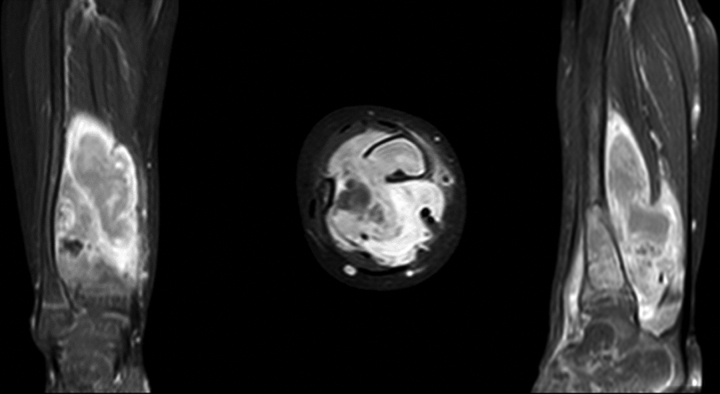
MRI (T1 with contrast) confirms the lesion in the distal tibia but reveals a huge soft tissue formation with consecutive bowing of the fibula with high contrast agent uptake. MRI = magnetic resonance imaging.
